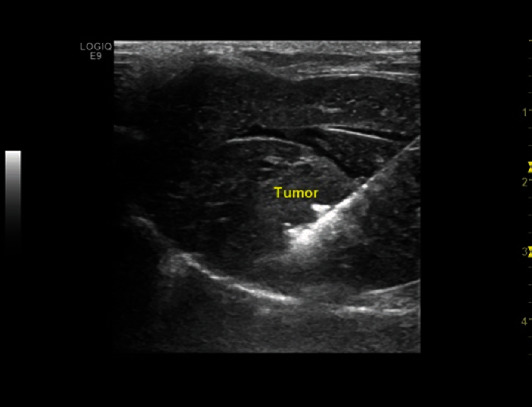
Microwave ablation of VX2 liver tumor in rabbits. A microwave ablation needle was inserted into the edge of the tumor nodule guided by ultrasound, ablating part of the tumor.
